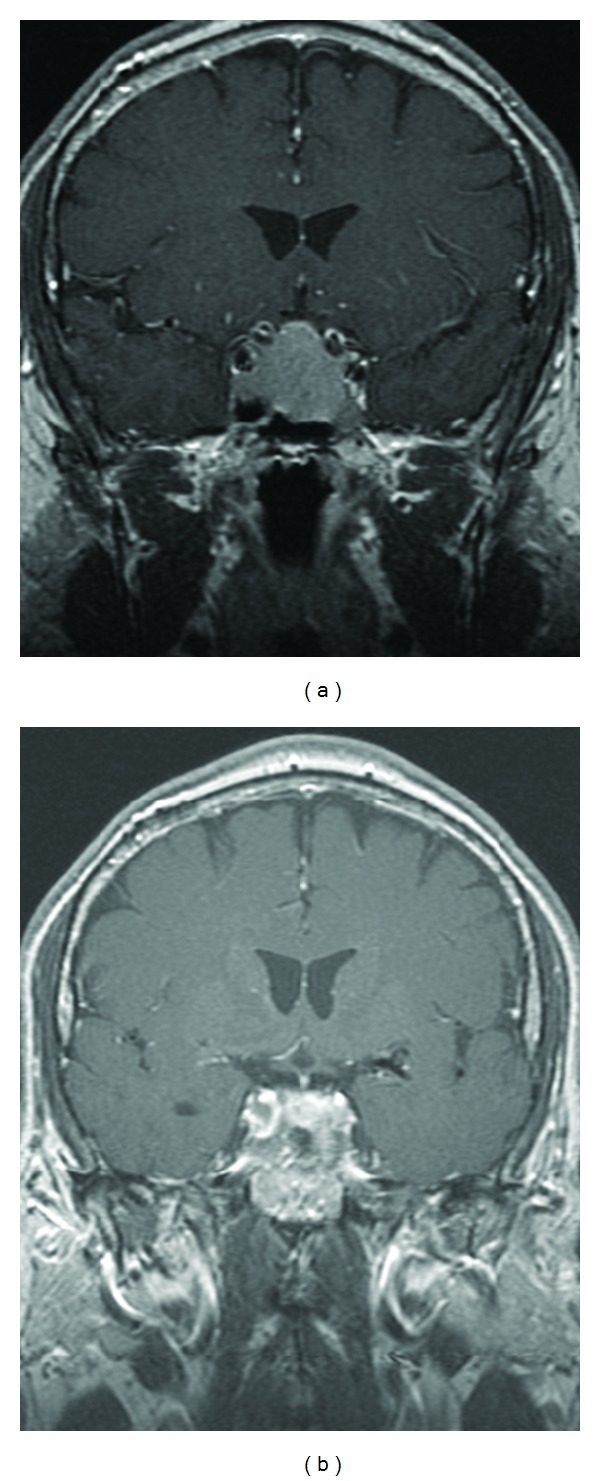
Pituitary MRI 2004. (a) Preoperative and (b) postoperative T1-weighted postgadolinium coronal images showing sellar tumor with suprasellar extension, mild mass effect on the optic chiasm, and extension into the sphenoid sinus. Postoperative image shows significant tumor debulking.
